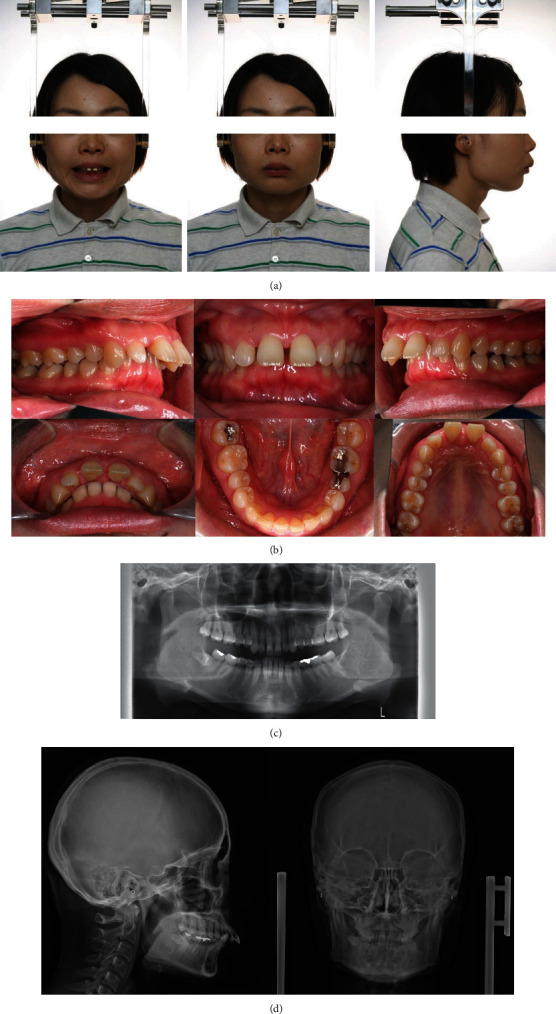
Pretreatment records. (a) Extraoral photographs. (b) Intraoral photographs. (c) Orthopantomograms. (d) Posteroanterior and lateral cephalograms.
